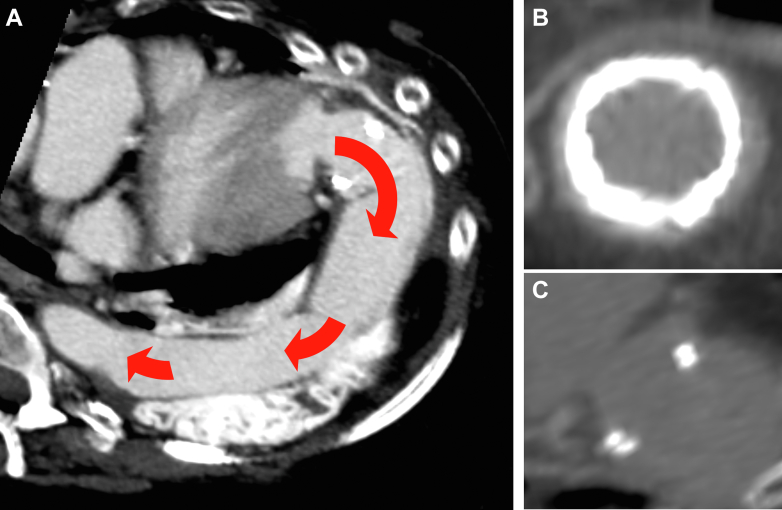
Follow-Up Imaging Follow-up computed tomography showed a good result and patency of the AAC with the absence of significant kinking (red arrows indicate blood flow) (A) and of subclinical conduit valve thrombosis (B and C).
